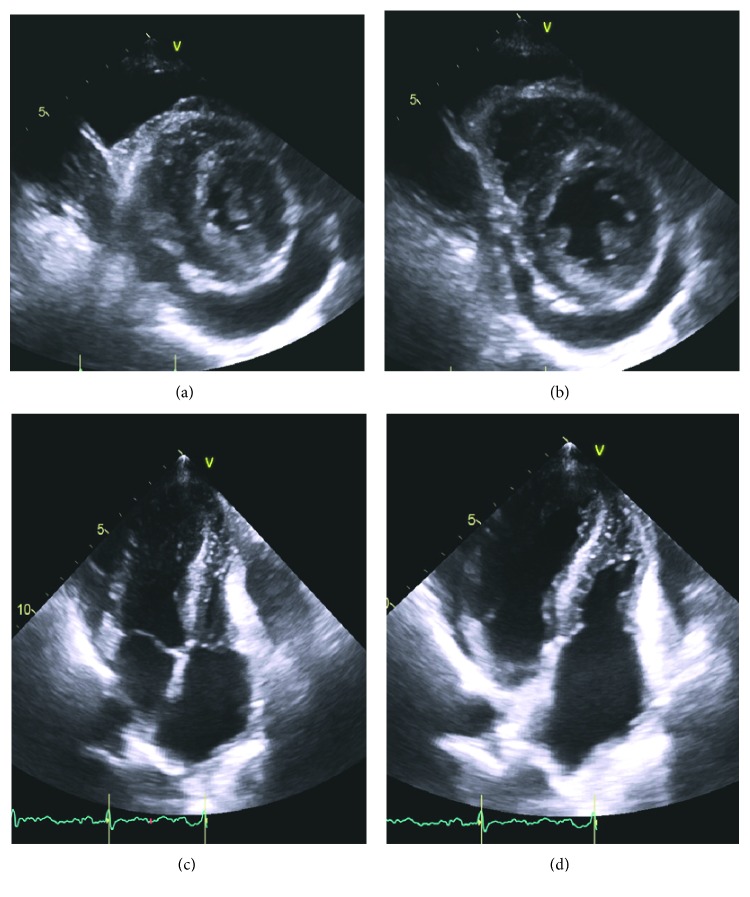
Transthoracic echocardiogram showing moderate-size pericardial effusion in both parasternal short axis view ((a) systole; (b) diastole) and parasternal long axis view ((c) systole; (d) diastole). Echocardiogram also demonstrates granular sparkling (speckled) appearance of myocardium, suggestive of cardiac amyloid.
